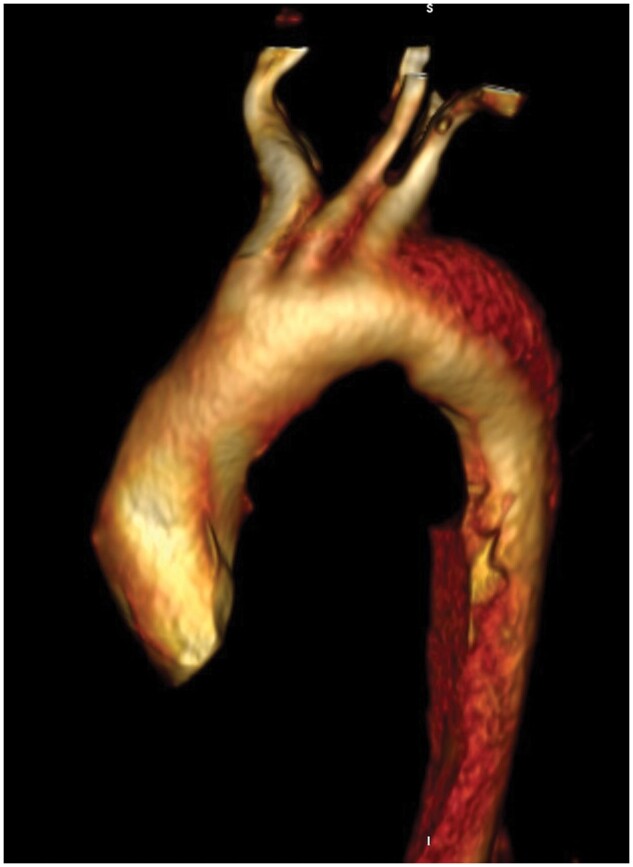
3-Dimensional computed tomography angiographic reconstruction shows an acute intramural aortic haematoma extending from the distal aortic arch to the abdominal aorta.
